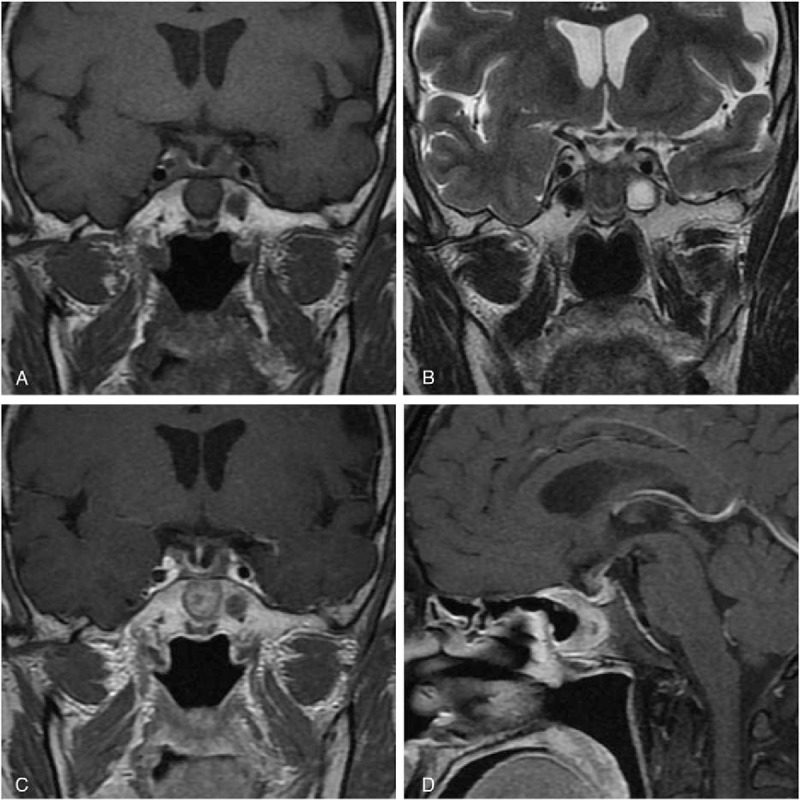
Postoperative MRI of showed the lesion after 3- month follow-up. (A) Coronal T1WI; (B) coronal T2WI; (C) coronal-enhanced T1WI; (D) sagittal-enhanced T1WI. MRI = magnetic resonance imaging, T1WI = T1-weighted image, T2WI = T2-weighted image.
